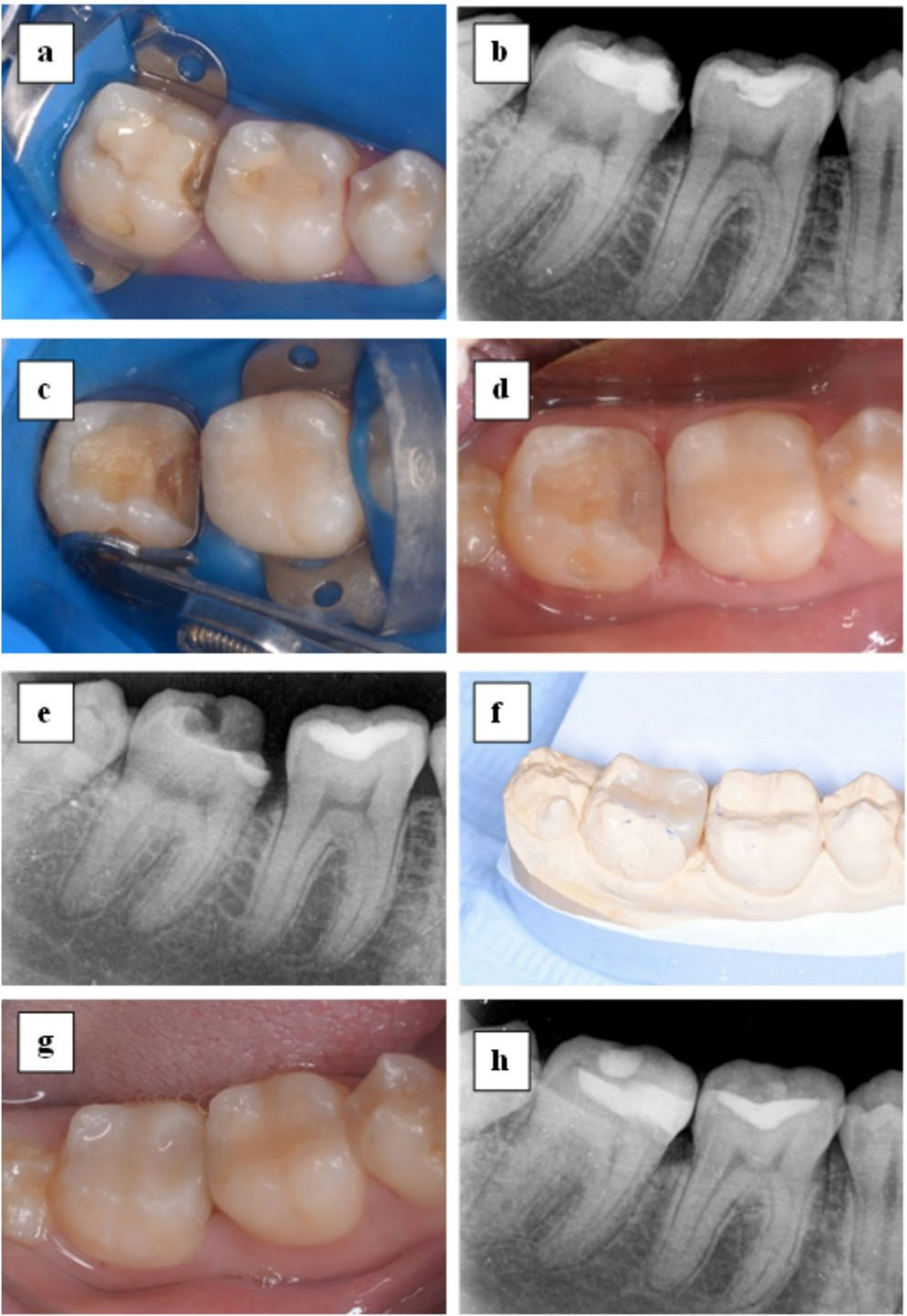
Composed figure representing the clinical procedures for tooth no. 47 a: preoperative photograph. b: preoperative radiograph. c: matrix application for deep margin elevation. d: Photograph after deep margin elevation. e: Radiograph after deep margin elevation. f: Nano-ceramic resin CAD/CAM onlay restoration. g: Photograph after onlay cementation. h: Radiograph after onlay cementation
